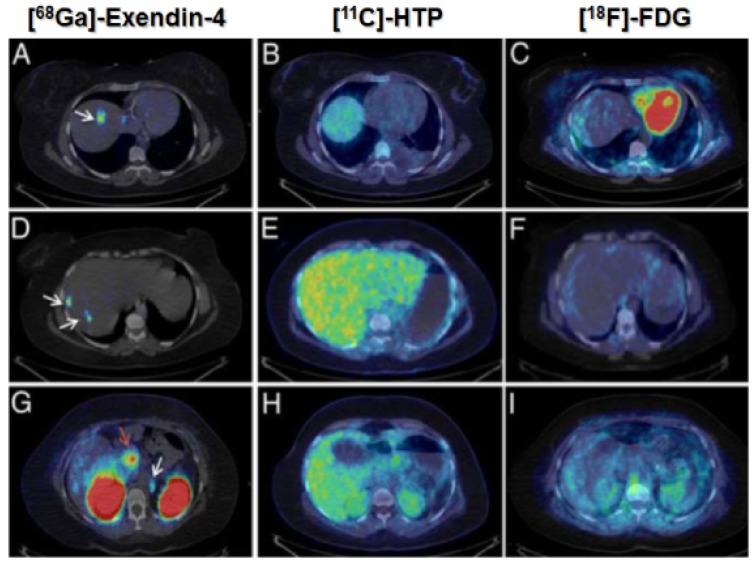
[^68^Ga]Ga-DO3A-Exendin-4/PET-CT revealed several GLP-1R positive lesions (white arrows) in the liver (A, D) and a paraortallymph node (G). Beta cells in normal pancreas (red arrow) have significant expression of GLP-1R and can also be visualized by this technique (G). No pancreatic or hepatic lesions could be detected by PET/CT using established tumor markers such as [^11^C]HTP (B, E, H) and [^18^F]FDG (C, F, I). Reproduced from 26.
